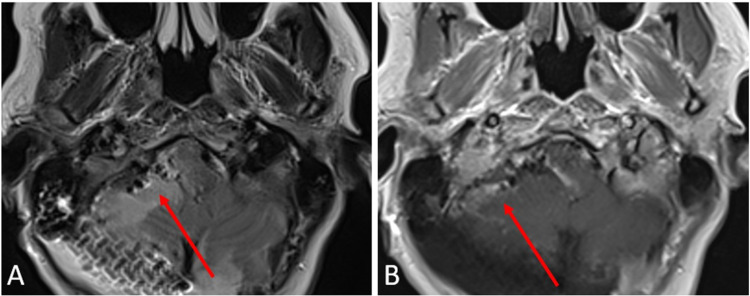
Postoperative imaging of a right posterior fossa calcifying pseudoneoplasm of the neuraxis (CAPNON). MRI - axial t2 (A) and axial t1 (B) status post-right suboccipital craniectomy with post-surgical changes in right cerebellar hemisphere. No evidence of residual posterior fossa mass. No evidence of residual enhancing mass.
